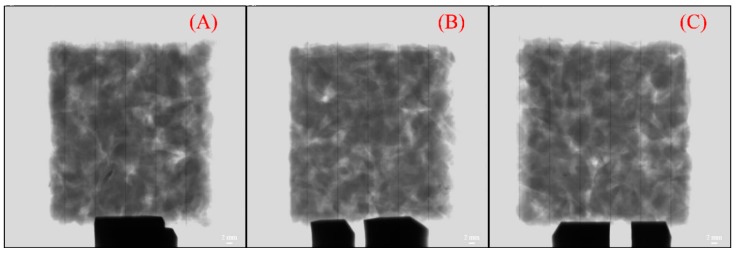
The CT images of cube samples with different voidage: (A) 4.08%, (B) 4.32%, (C) 4.56%.
